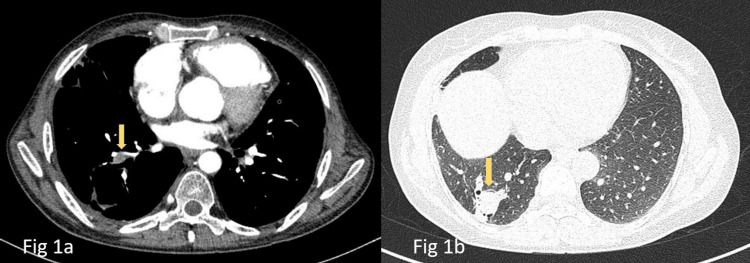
Aspergilloma in a patient of pulmonary thromboembolism (a) Computed tomography of the chest (mediastinal window) shows filling defect in the right basal pulmonary artery (downward arrow). (b) Computed tomography of the chest (parenchymal window) just a section below shows the same cavity with a meniscus sign suggestive of Aspergilloma (downward arrow).
